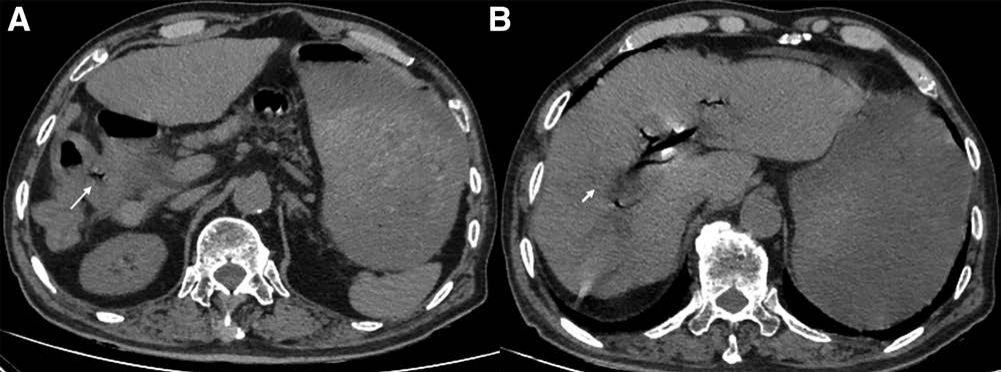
Biliary-enteric fistula in a 74-year-old-man. (A) CT scan in the axial plane demonstrates a communication between gallbladder and duodenum (arrow) suggesting biliary-enteric fistula. (B) CT scan in the axial plane shows gas in the gallbladder (short arrow). CT = computed tomography.
